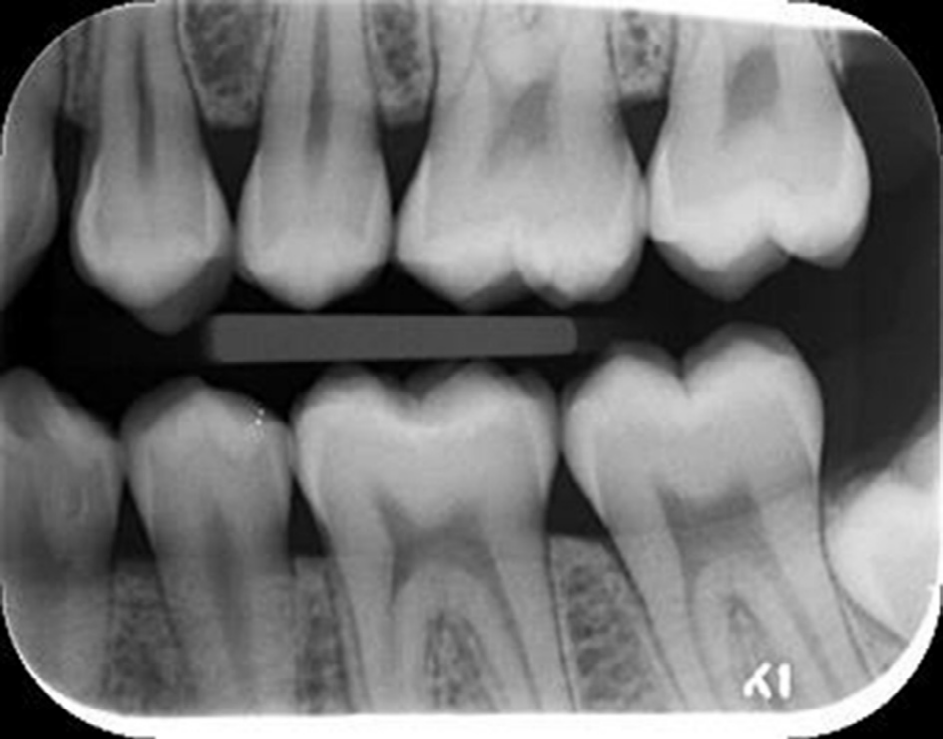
Example of a BW with eight included teeth (first and second upper premolars and molars, first and second lower premolars and molars). Tooth 34 has an enamel defect, which is too coronally positioned to be mistaken for ECR. BW, bitewing; ECR, external cervical resorption.
